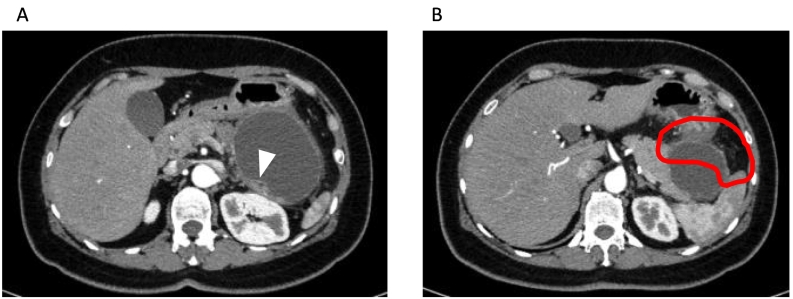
A/B; CT. The contrast-enhanced CT revealed a unilocular cystic lesion in pancreatic tail sized in 80 mm which had partial thicken wall (arrow head). There were disproportionate fat stranding around cystic lesion (in circle).
